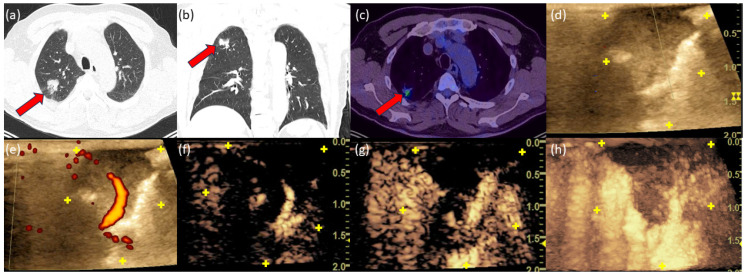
Chest CT scan in axial view (a) and coronal view (b) showing a suspicious tumor in the upper lobe (red arrows). FDG-PET/CT revealed an SUV of 4.8 (c). In B-mode, the dimensions of the tumor can be seen (d). The yellow “+” marks the tumor margin. (e) The power Doppler with central and peripheral vessel sections. In CEUS (f) at t = 9 s, the central and peripheral contrast agent uptake can be seen, which increasingly spreads throughout the tumor over time (g) at t = 14 s. (h) The 3D reconstruction of the CEUS confirmed a marginal and central contrast center uptake.
